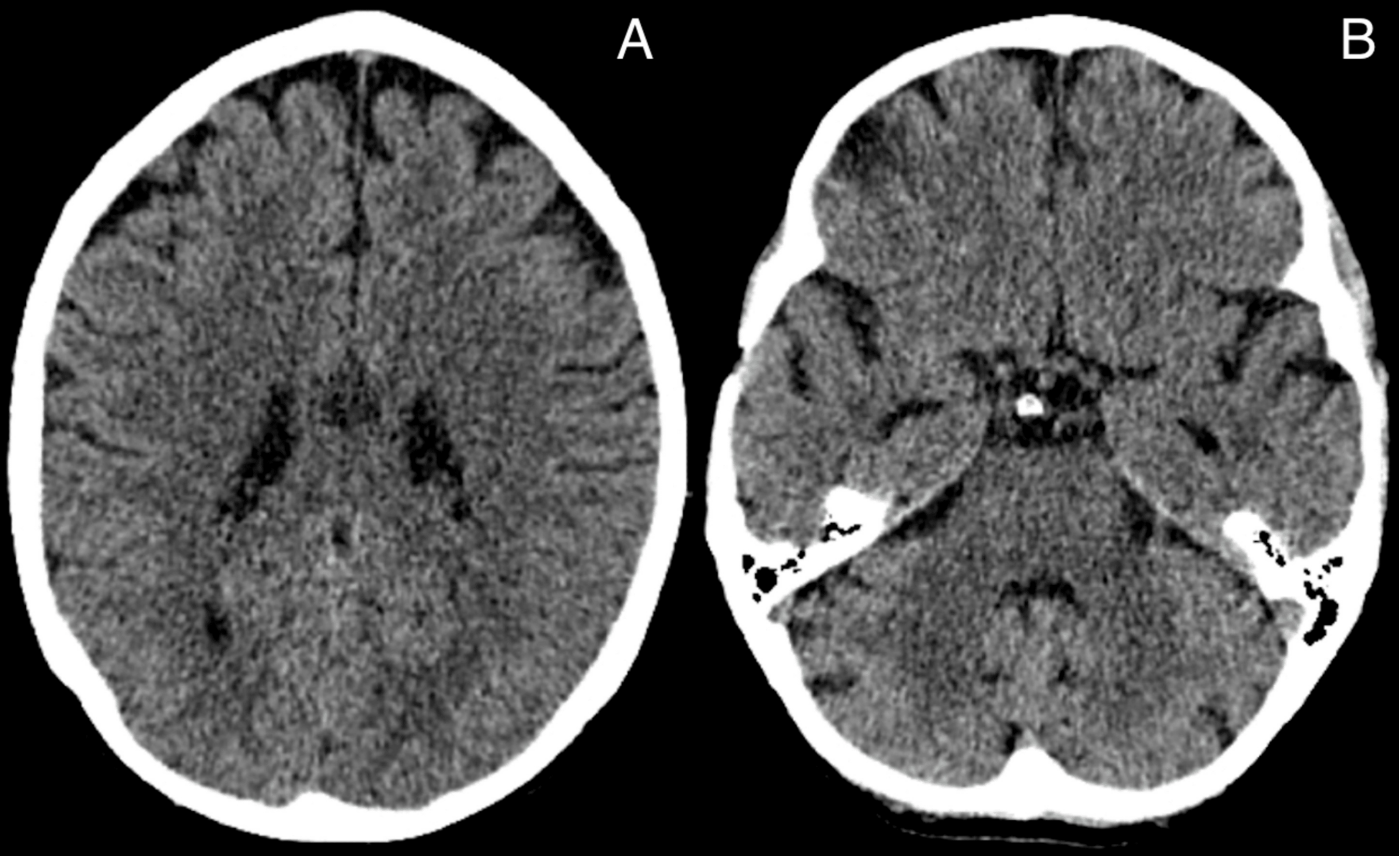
Non-contrast CT brain images showing no evidence of acute intracranial pathology. A: axial plane, demonstrating no evidence of acute intracranial pathology at the level of the cerebral cortex and basal ganglia. B: axial plane, demonstrating no evidence of acute intracranial pathology at the level of the tentorium cerebelli.
